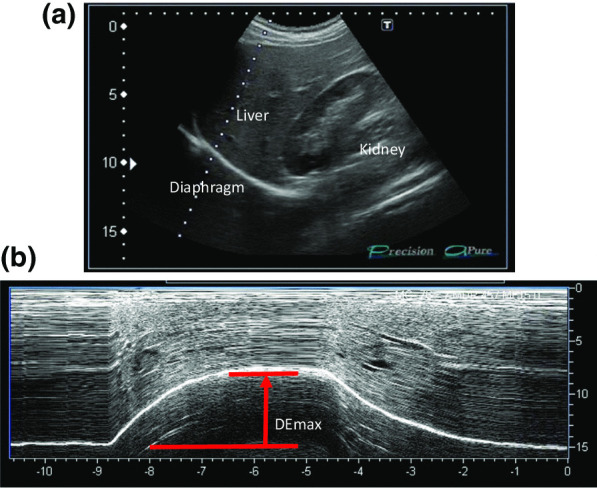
Representative image of the right diaphragm. The probe was positioned below the right costal margin between the midclavicular and anterior axillary lines. A Two-dimensional ultrasonographic image of the right hemidiaphragm (B-mode). Diaphragmatic movements were recorded in M-mode during deep breathing (DE_max_) (B)
